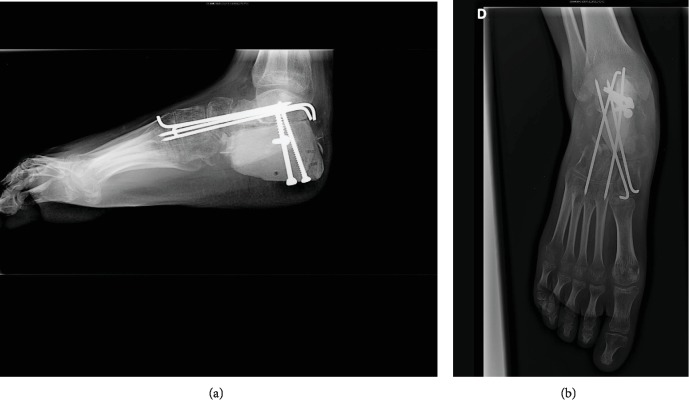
Lateral (a) and anteroposterior (b) radiographs of the right calcaneus. Postoperative result at 3 months of the resection of the right calcaneus with a reconstruction by iliac graft along with a triple arthrodesis of the hindfoot.
